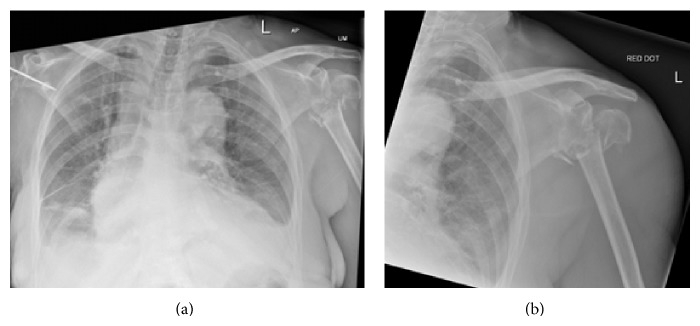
AP radiographs of the left shoulder showing a proximal humeral fracture (a, b).
